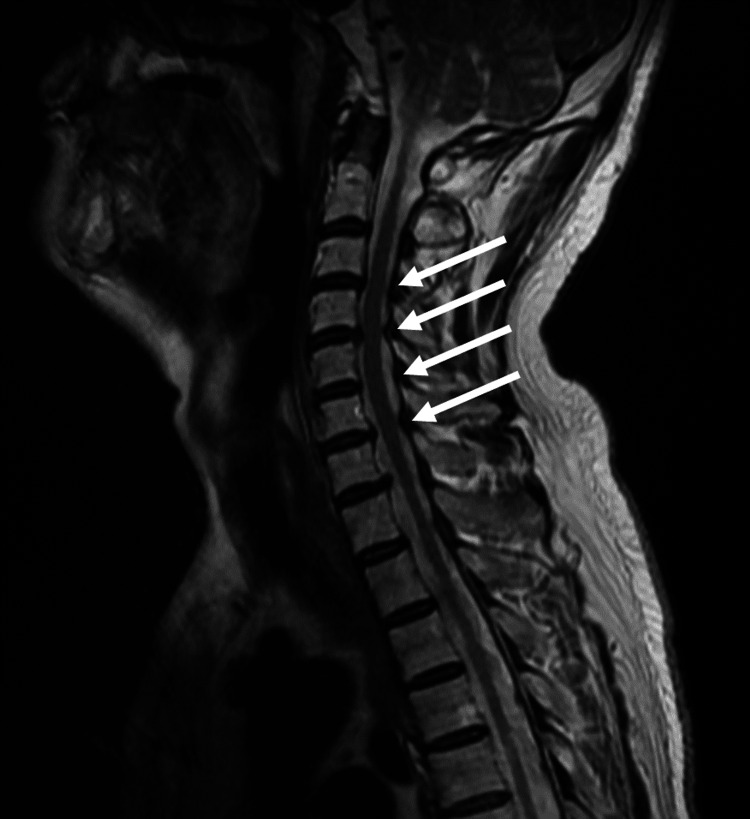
Cervical spine MRI demonstrating cervical spondylosis. Cervical spine MRI scan reveals osteophytes from C3/4 to C7/T1 levels and posterior protrusion of intervertebral discs, causing compression of the dural sac. These findings are consistent with cervical spondylosis.
